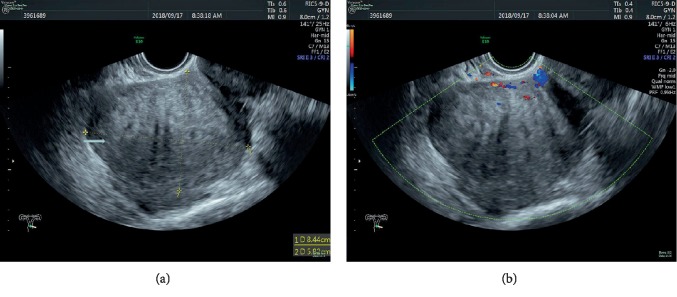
(a) Hypoechoic mass lesion on ultrasound image. (b) Strip-like blood flow signal of the mass on color Doppler flow image.
